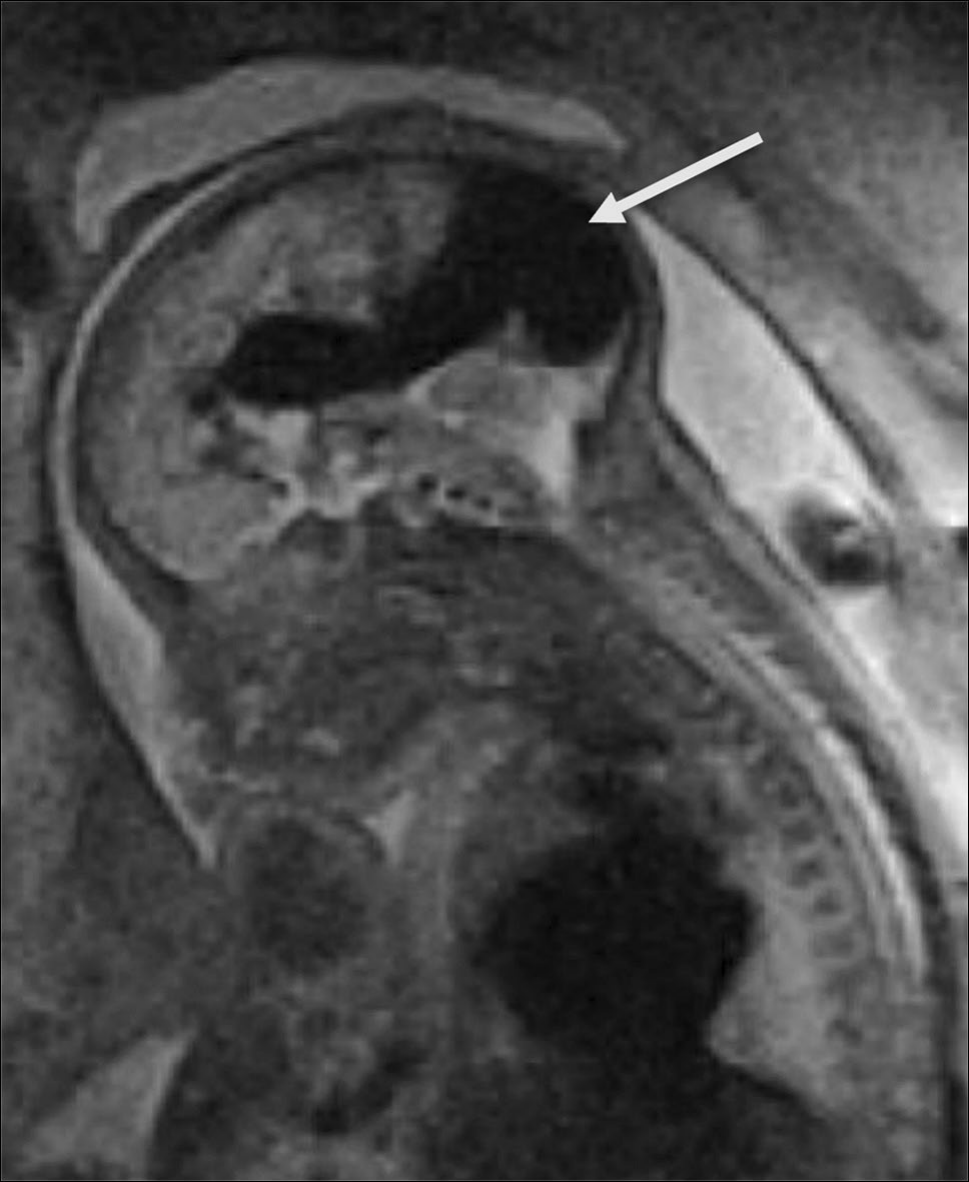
Vein of Galen malformation. Fetal magnetic resonance imaging in a 23-week gestational age male fetus with a vascular, midline posterior supratentorial structure that showed turbulent flow on color Doppler ultrasound (not shown). Sagittal T2-weighted image confirms the presence of an aneurysmal flow void along the course of internal cerebral veins and, more prominently, of the straight sinus (*arrow*) in keeping with Vein of Galen malformation
